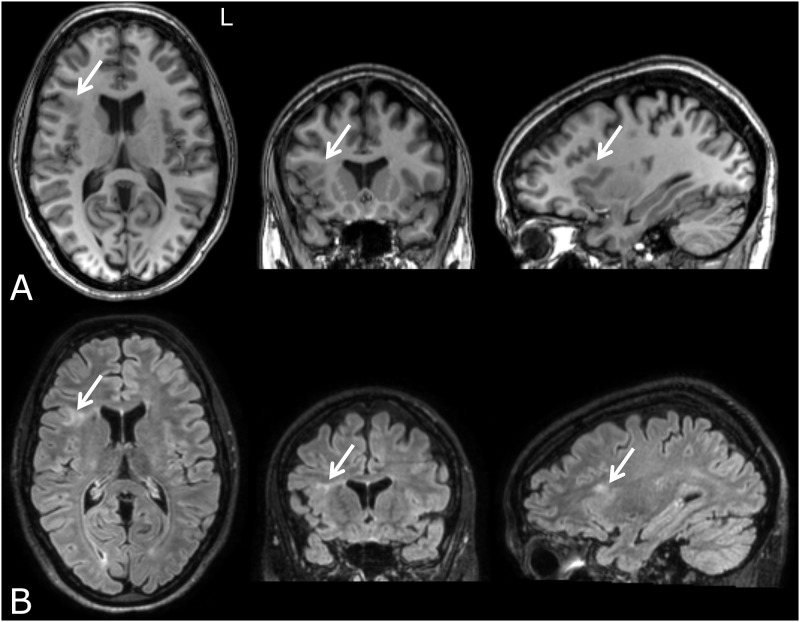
Conventional structural MRI features of focal cortical dysplasia in a representative subject (patient 1) for 3 orthogonal slices. The abnormalities are indicates by arrows; in the T1-weighted images (A) these are cortical thickening and blurring of the gray matter-white matter interface; in the FLAIR-weighted images (B), hyperintensities in the underlying white matter can be observed.
